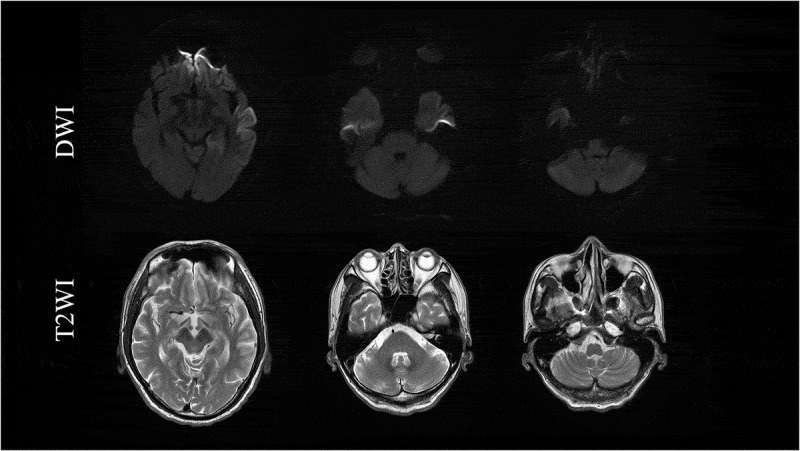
Magnetic resonance imaging findings in T2-weighted images (T2WI) and diffusion-weighted images (DWI) in the brainstem. Normal findings were observed.
